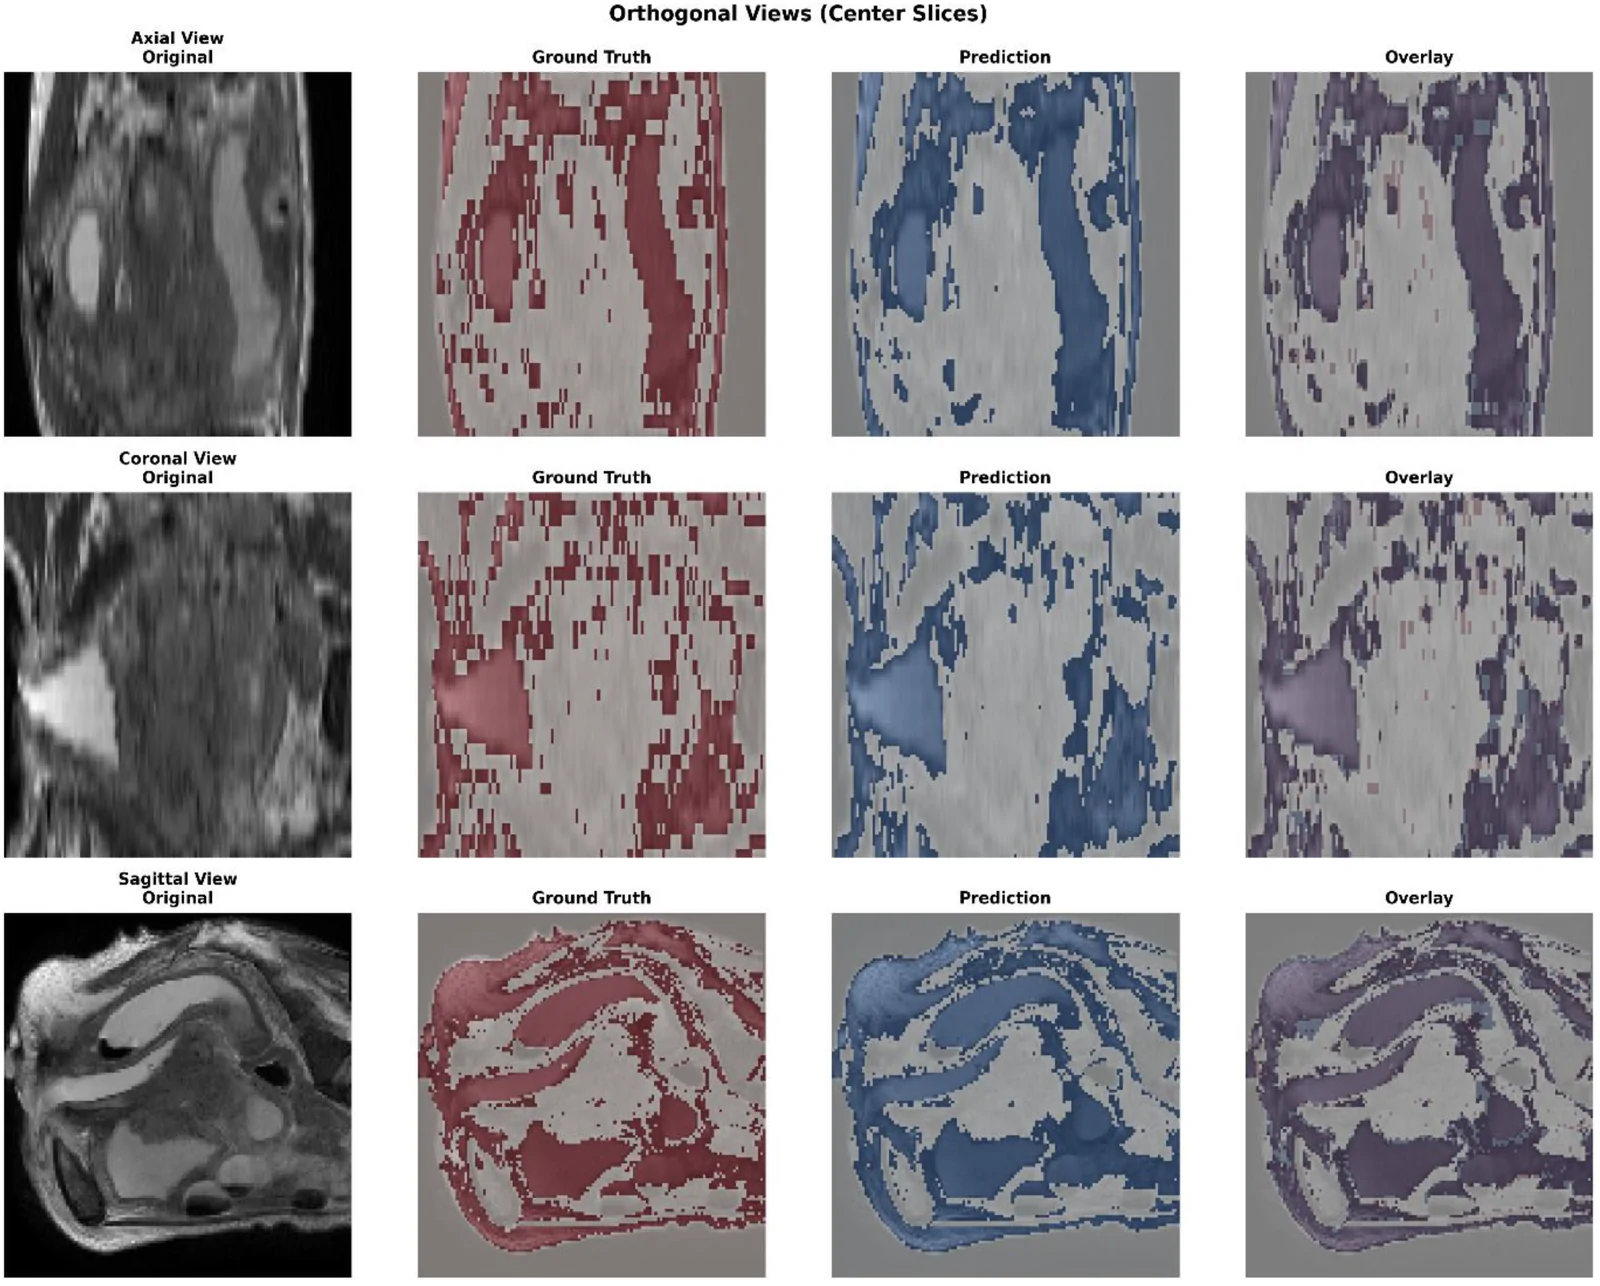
Orthogonal plane visualization of cervical tumor segmentation on T2-weighted pelvic MRI.
